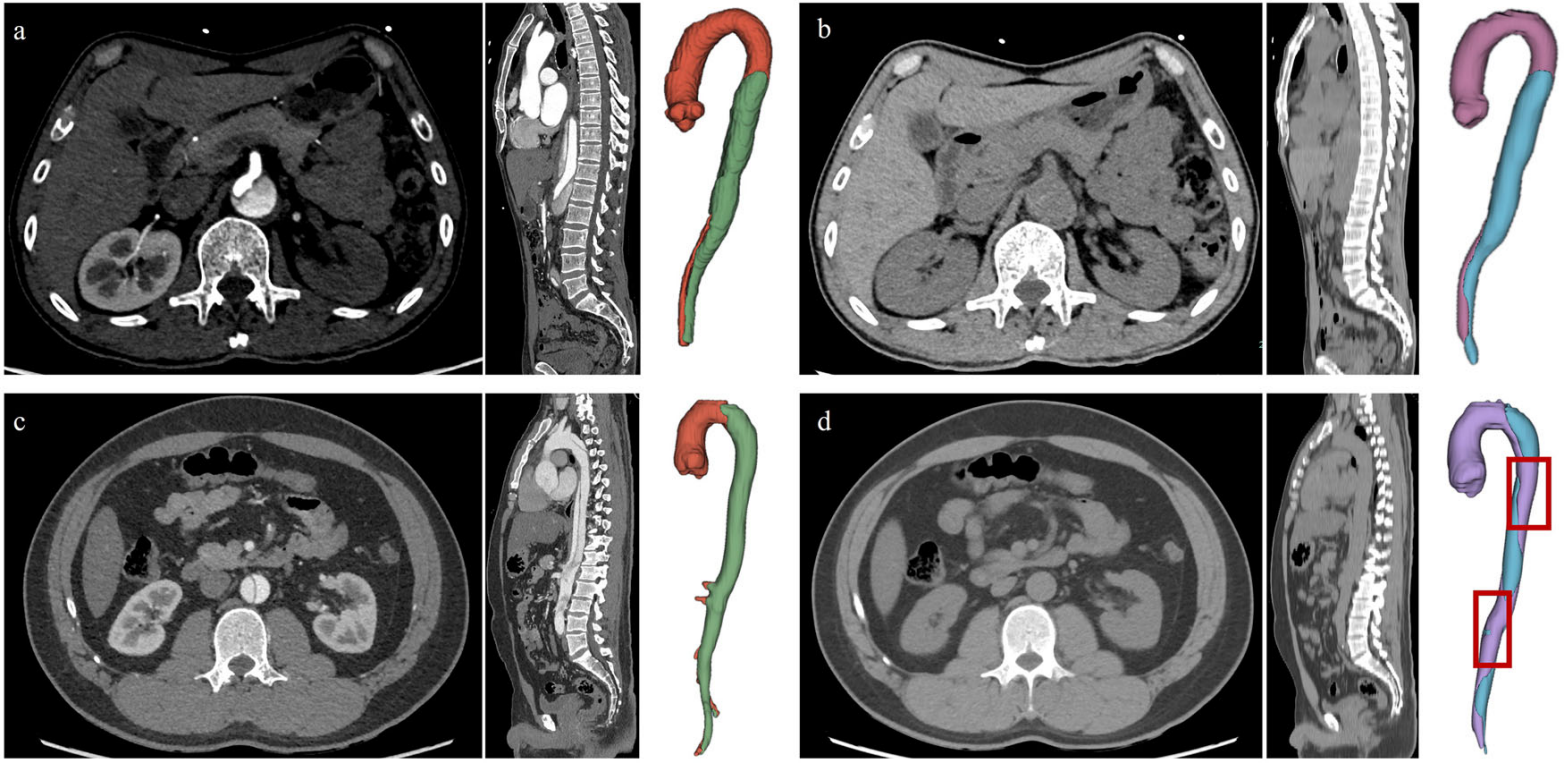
The examples of good and bad segmentation cases for AD. a, b A 64-year-old man, admitted to the hospital with back discomfort for one month. The CTA images and the 3D-volume reconstruction (VR) view of the aorta show the dissection of the aorta (a). The non-contrast images at the same level and the 3D-VR show good segmentation of true/false lumens on non-contrast images (b). The ground-truth false lumen volume is 107,282 mm^3^ and the predicted false lumen volume is 134,885 mm^3^. The Dice coefficient is 0.817. c, d A 33-year-old man, admitted to the emergency department with severe chest pain for 10 min. The 3D-VR of NCCT images presents a not-satisfactory segmentation (d compared with c). The standard false lumen volume is 155,658 mm^3^ and the predicted false lumen volume is 97,159 mm^3^. The Dice coefficient is 0.580
